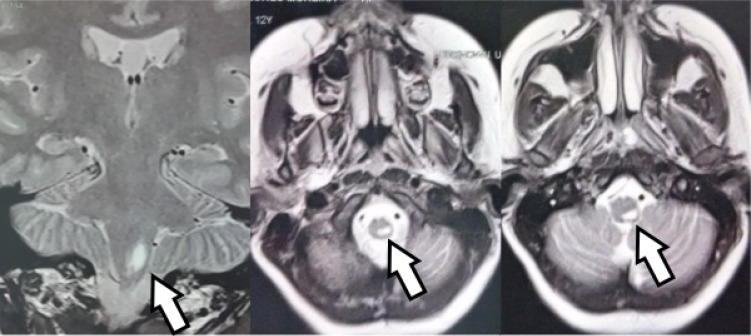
Brain resonance shows lesions in the brainstem and area postrema. Coronal and axial sections with T2-weighted acquisition.
